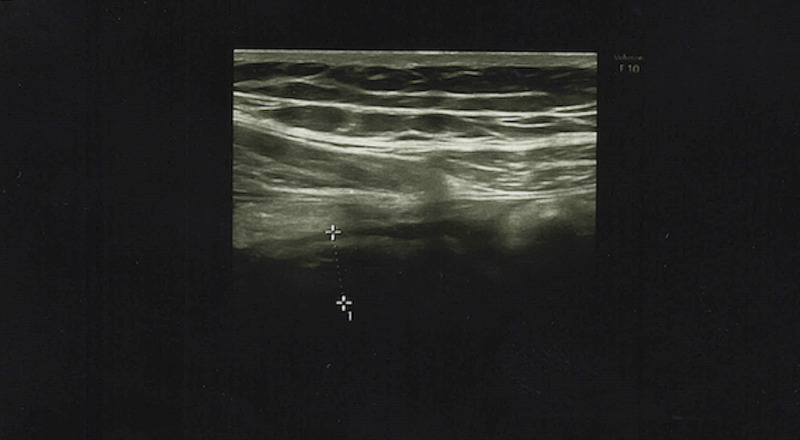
Ultrasound showing fluid in the ileocecal fossa and a 10 mm appendiceal diameter with periappendiceal inflammation
